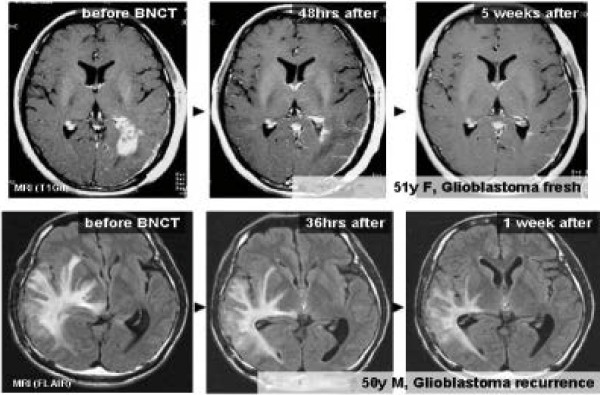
Radiographic changes following BNCT in two representative patients with GBM. In both, there was a reduction in both mass and peritumoral edema without the administration of corticosteroids or mannitol within a few days. This is also shown in the FLAIR image of Case #12.
